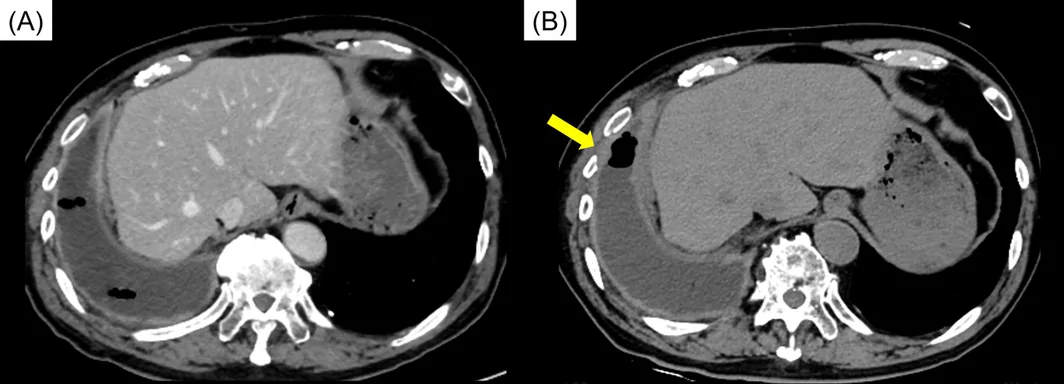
(A) Chest CT before surgical biopsy (June 2025) showing diffuse right pleural effusion and pleural thickening, but no obvious mass formation. (B) Chest CT performed 2 months after the surgical biopsy (August 2025), revealing a new mass with bone invasion in the right 8th rib (arrow).
